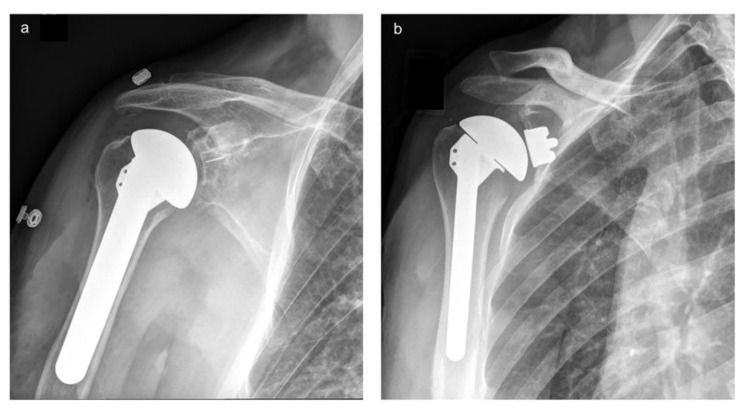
Radiographic images demonstrate an anatomic total shoulder arthroplasty of a right shoulder with part (a) a cemented polyethylene (PE) and part (b) a trabecular metal (TM) glenoid.
